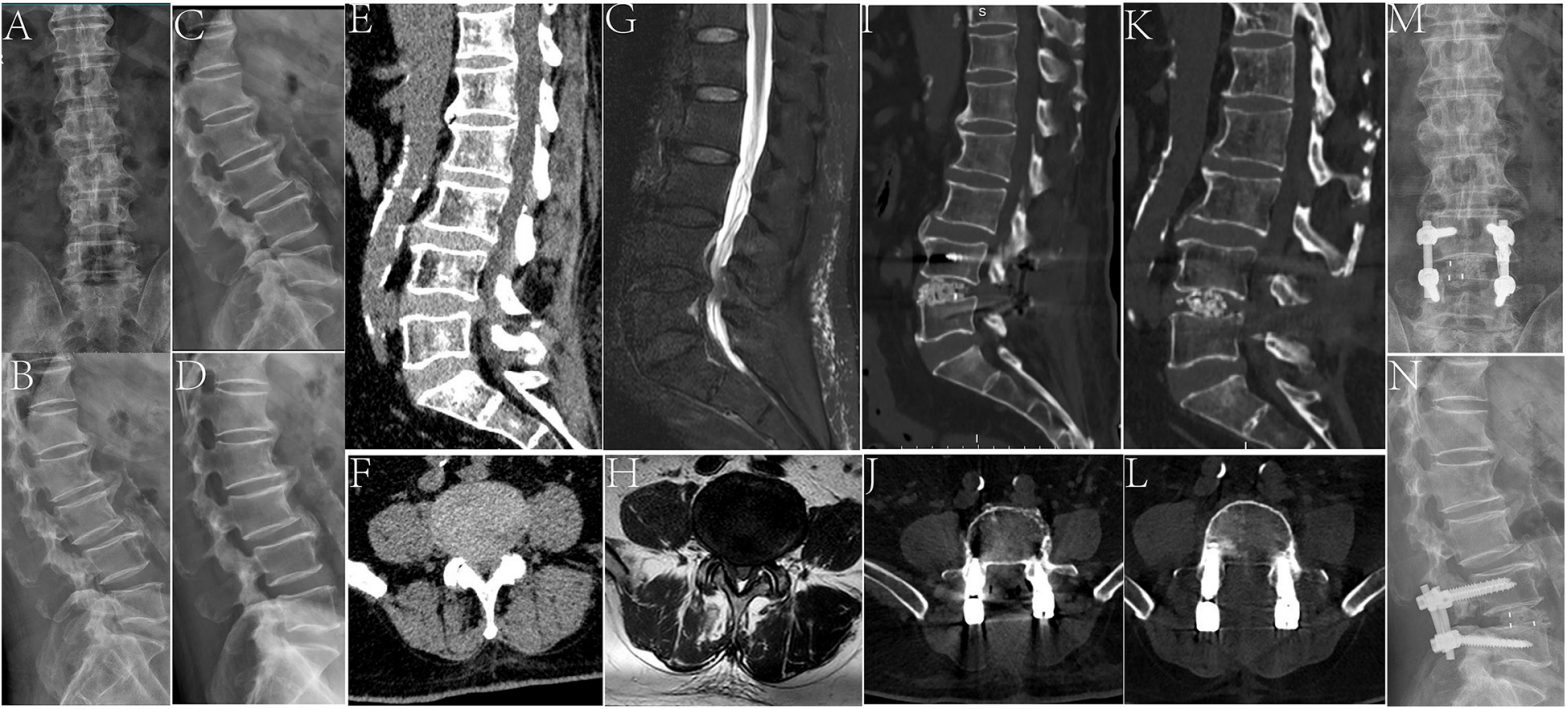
An 80-year-old male patient who underwent PLIF surgery. (A,B) Preoperative lumbar lateral x-ray. (C,D) Preoperative lumbar imaging in the dynamic position. (E,F) Preoperative lumbar MRI T2 images in the sagittal and transverse planes showed L4/5 spinal stenosis. (G,H) Preoperative lumbar CT in the sagittal and transverse positions. (I,J) Postoperative lumbar CT in the sagittal and transverse positions showed that the spinal canal was completely decompressed and the screw was fixed in position. (K,L) The last follow-up lumbar CT images in the sagittal and transverse positions. (M,N) Anteroposterior and lateral x-ray images at the last follow-up.
